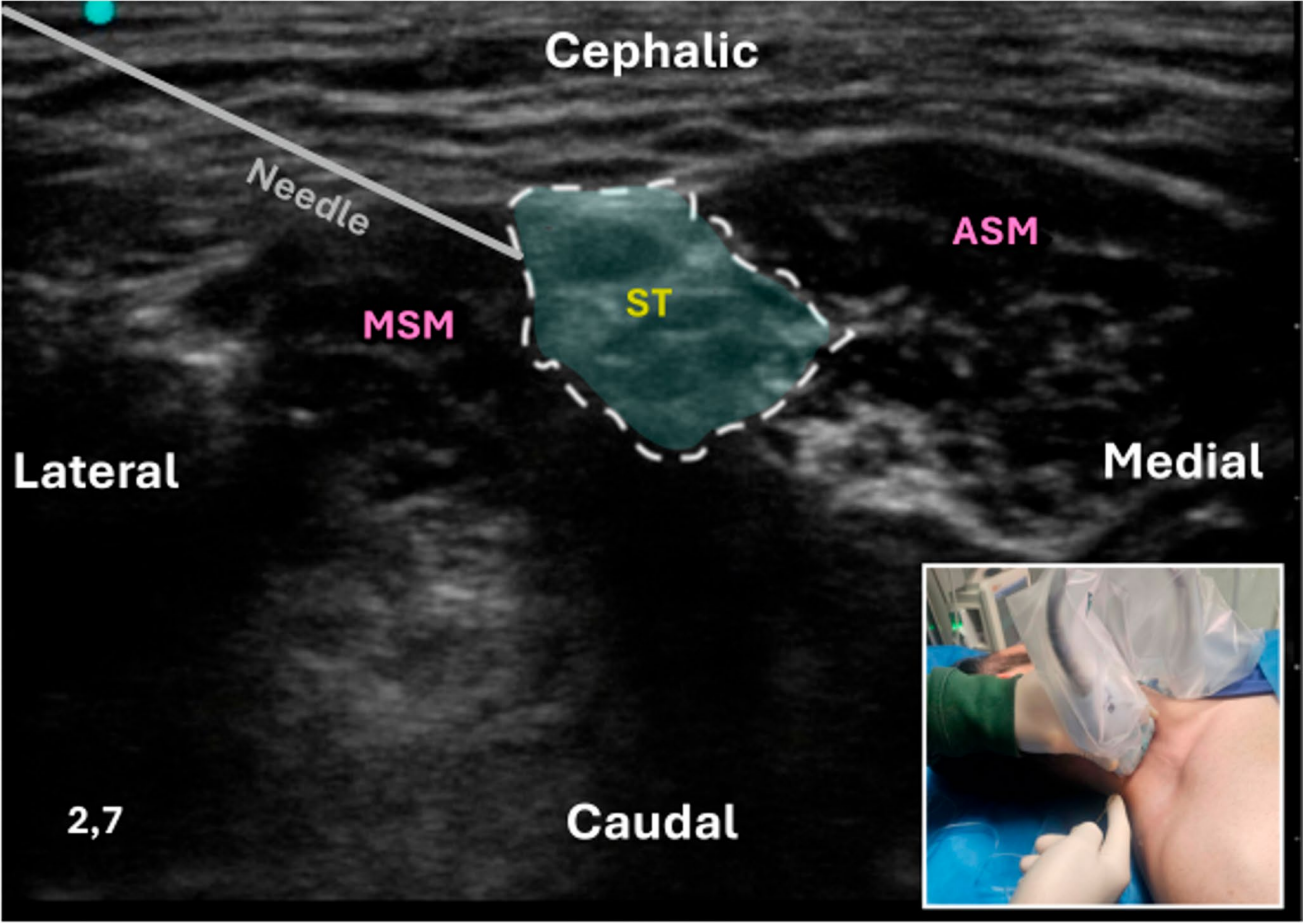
Ultrasound-guided STB. The block is performed at a lower level than the interscalene block, identifying the superior trunk (outlined by the white dashed lines), formed by fusion of C5 and C6 nerve roots and located between the anterior scalene (ASM) and middle scalene (MSM) muscles. The needle is advanced in-plane from lateral to medial. The blue-shaded area within the dashed lines represents the spread of local anesthetic around the superior trunk. The inset shows the corresponding transducer and needle position
